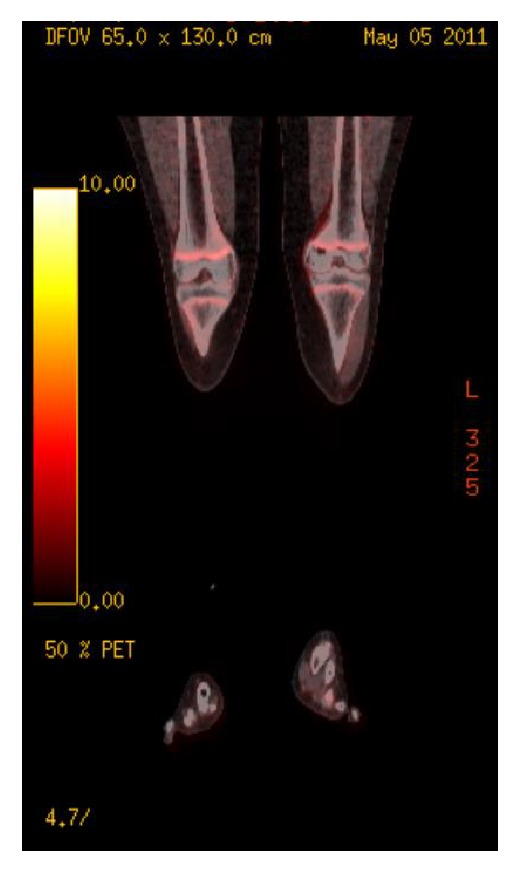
Fludeoxyglucose-positron emission tomography (FDG-PET), May 2011. Non-FDG-avid mixed sclerotic and lytic lesion of the left medial femoral condyle, consistent with resolved lymphoma.
